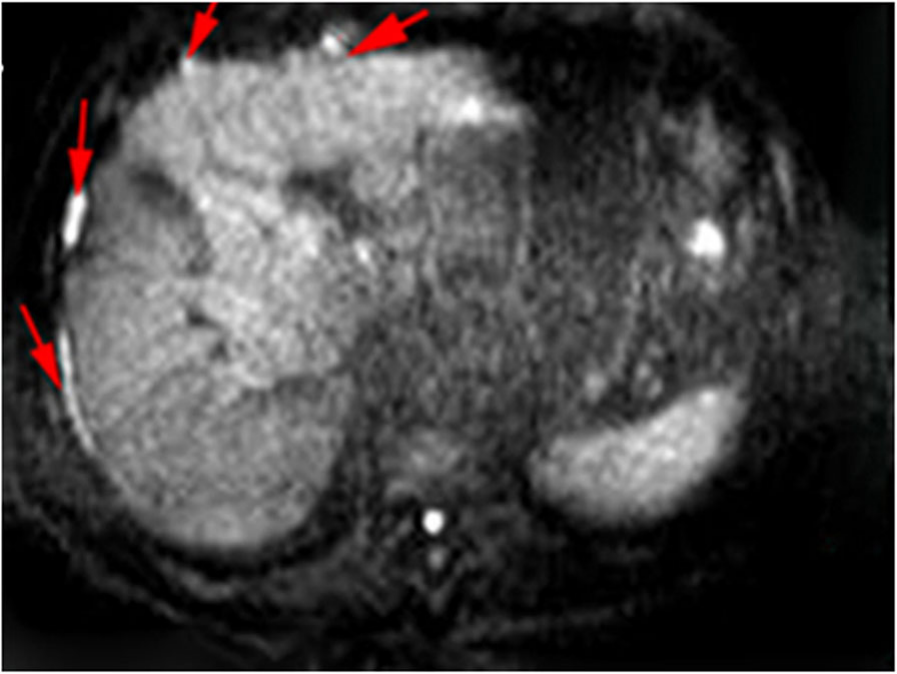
Abdominal enhanced magnetic resonance image analysis showed multiple nodule-like lesions in the hepatic capsule (*red arrows*)
